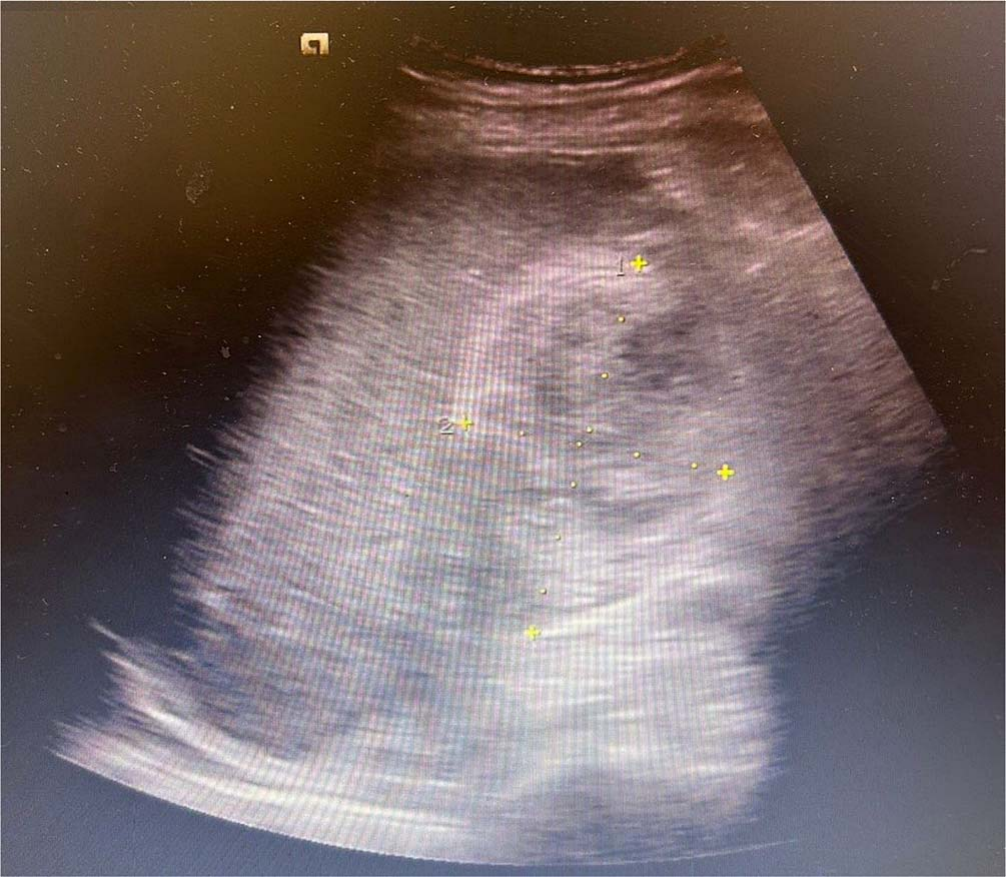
Ultrasound abdomen showing oedematous gallbladder with a heterogenous hypoechoic collection in gallbladder fossa (approximately measuring 9 × 4 cm).
